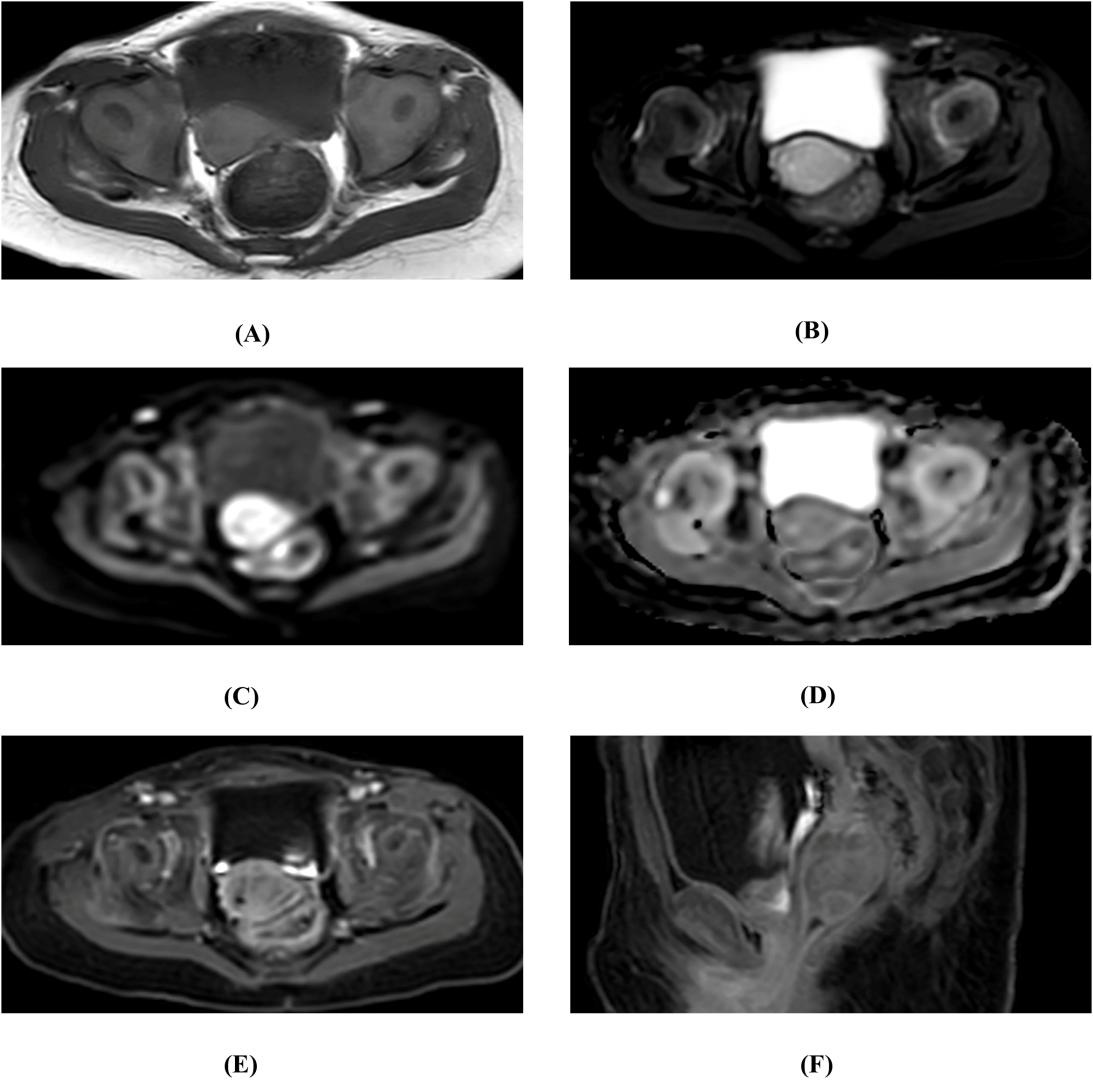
The results of the preoperative examination. (A) Axial T1-weighted image (T1WI). (B) Axial fat-suppressed T2-weighted image (FS T2WI). (C) Axial diffusion-weighted image (DWI; b = 800 mm^2^/s). (D) Axial apparent diffusion coefficient (ADC) map. (E) Axial post-contrast fat-suppressed T1WI. (F) Sagittal post-contrast fat-suppressed T1WI. Fat-suppressed T2WI shows a vaginal tumor with slightly heterogeneous high signal intensity. On high-b-value DWI, the solid-enhancing part of the tumor exhibits high signal intensity, with a low ADC (ADC mean = 1.2×10–^3^ mm^2^/s). The mass shows mild to moderate enhancement on post-contrast fat-suppressed T1WI, with enhancement lower than that of the adjacent vaginal tissue.
